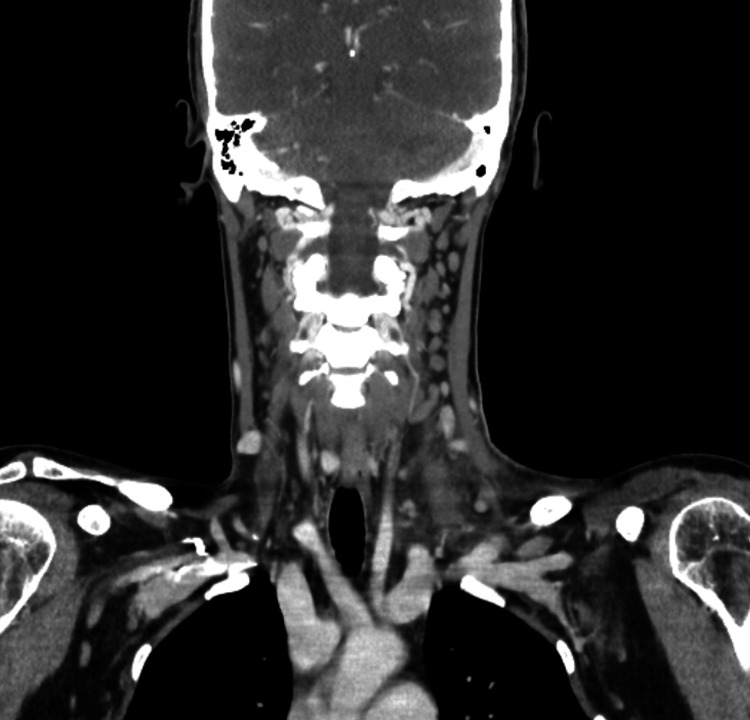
Coronal head and neck computed tomography with contrast Coronal head and neck computed tomography showing induration in the left lower neck and subclavicular fossa, extending into the left scalene triangle indicating cellulitis and myositis. Multiple enlarged reactive lymph nodes are appreciated. There is no evidence of abscess, fluid collection, or cervical spondylodiscitis.
